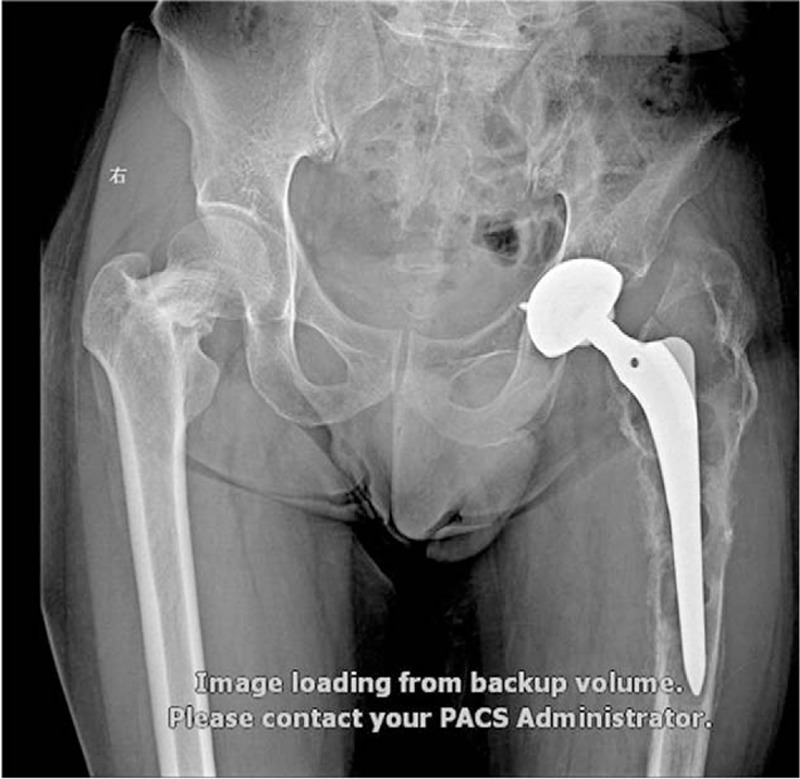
X-ray image before the second RTHA on the left side and before the third THA on the right side. RTHA = revision total hip arthroplasty, THA = total hip arthroplasty.
